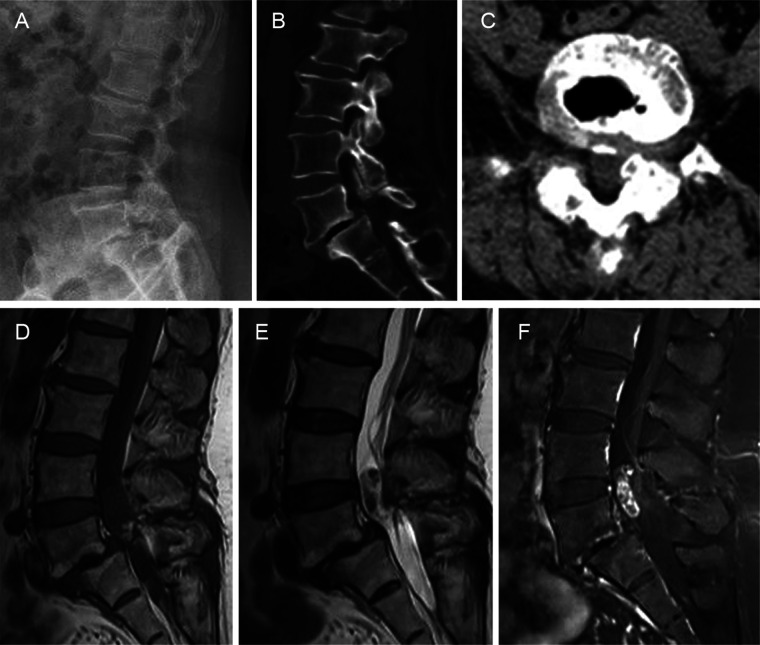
Preoperative imaging. (A) Radiographs of the lumbar spine showing grade II L4–5 spondylolisthesis. (B,C) Computed tomography images showing left L5–S1 intervertebral foramen stenosis and the isthmic fracture. (D,E) Magnetic resonance image shows equal or low signals on the T1-weighted imaging sequence and high signals on the T2-weighted imaging sequence. (F) Enhanced scan showing inhomogeneous enhancement.
